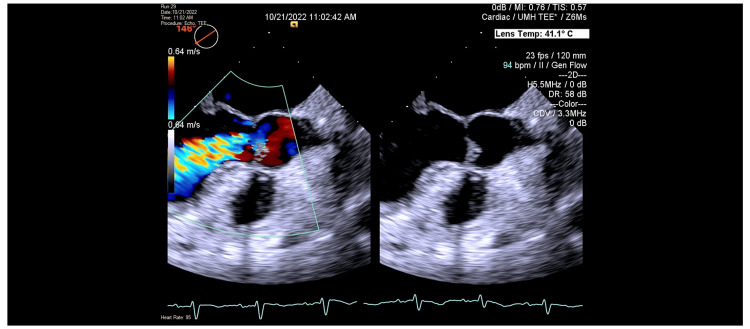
Long axis view of Transesophageal Echocardiogram demonstrating central jet regurgitant flow despite mobile anterior leaflet, suggestive of perforation.
